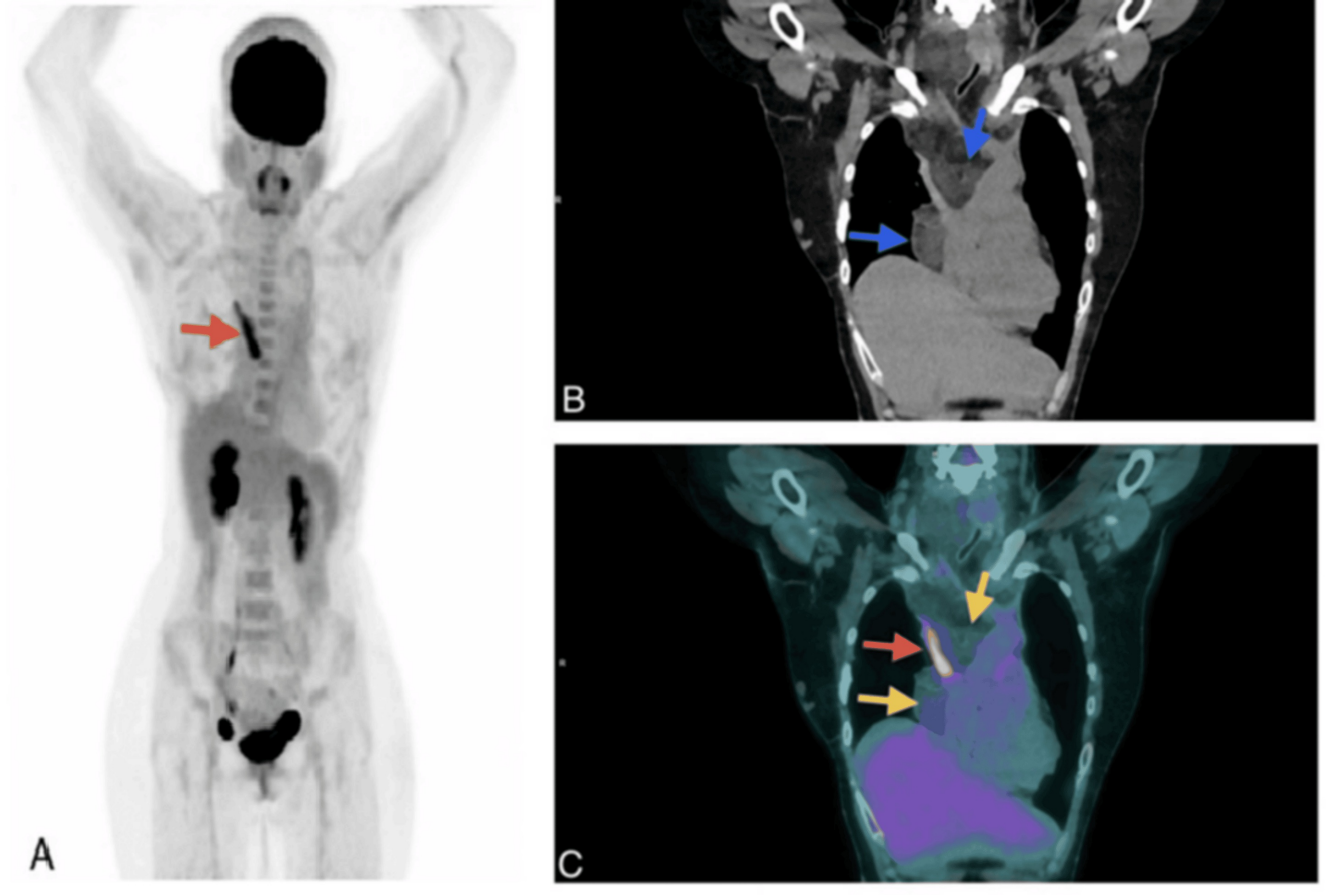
(A) FDG-PET/CT whole-body MIP showing hypermetabolism along the SVC (red arrow). (B) Coronal CT (soft-tissue window) showing a well-defined, multilobulated mediastinal mass with predominantly fat density (blue arrows). (C) Coronal PET/CT fusion showing alternating normometabolic and mildly hypermetabolic areas (yellow arrows) (SUVmax 2.54) and marked hypermetabolism (SUVmax 7.09) along the SVC (red arrow), associated with an endoprosthesis FDG-PET/CT: fluorodeoxyglucose positron-emission tomography computed tomography; MIP: maximum intensity projection; SVC: superior vena cava
